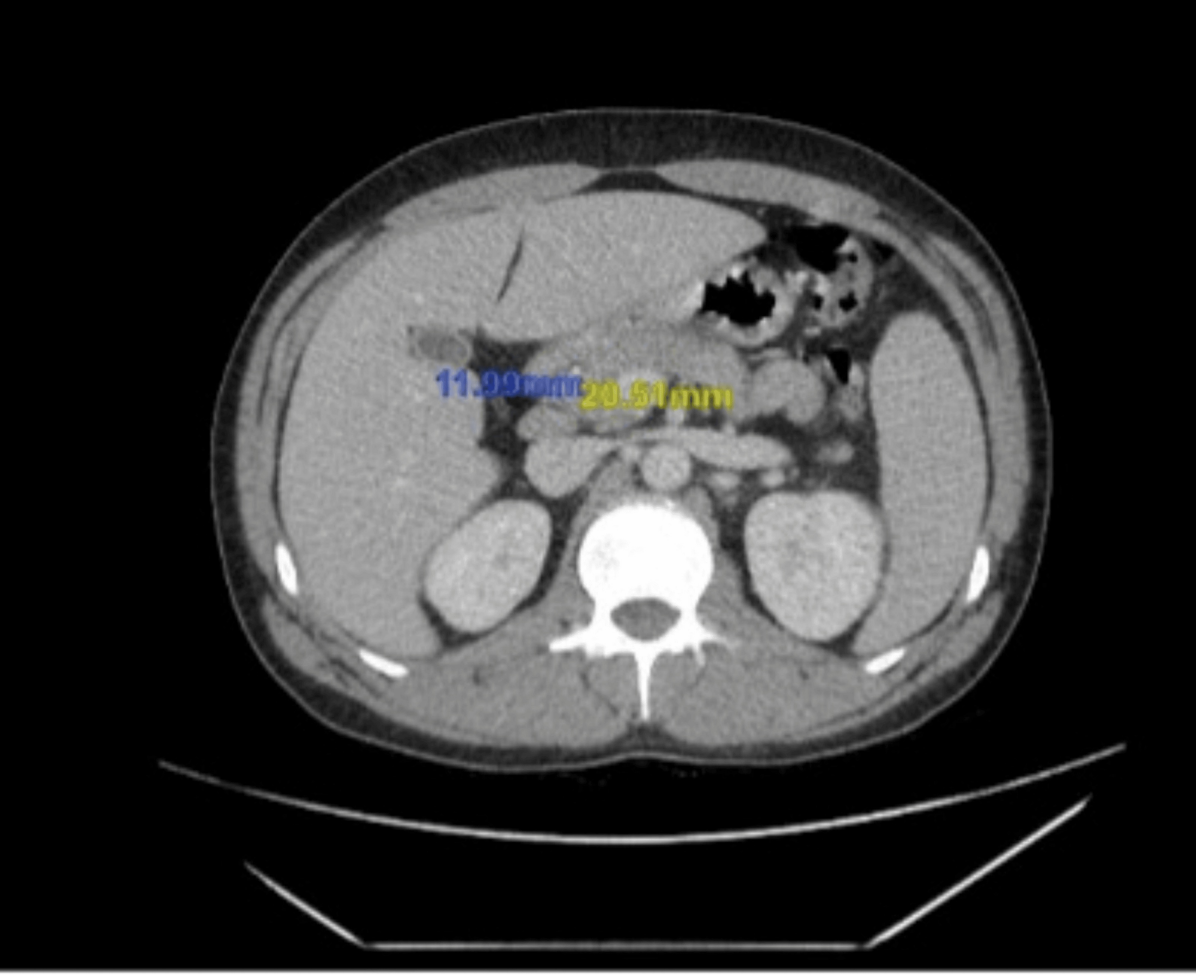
Computed tomography of the abdomen and pelvis demonstrating enlarged periportal and portocaval lymph nodes, as marked by the yellow and blue numerical values
